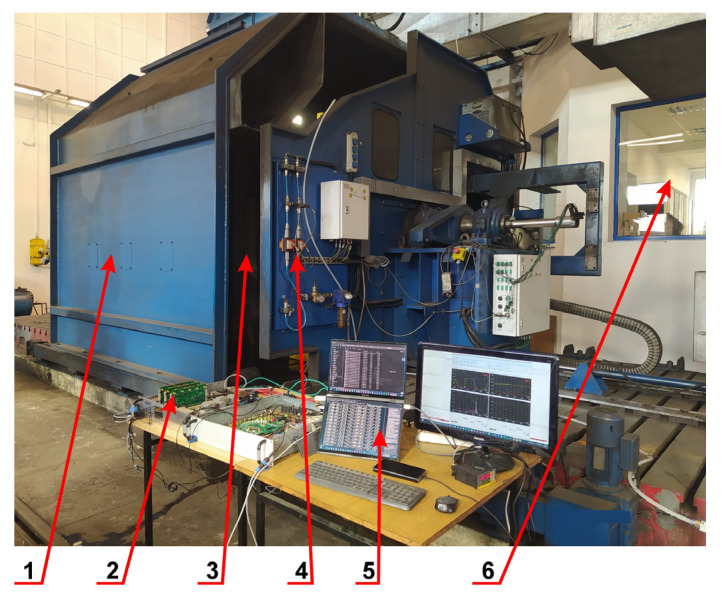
The test rig adopted for the high-speed flaw detection testing. 1—Drive system, 2—Measurement apparatus with a multichannel ultrasonic flaw detector; 3—Measuring chamber with the test wheel; 4—System for supply and measurement of the coupling fluid; 5—Computerized control and recording unit of the ultrasonic apparatus; 6—Control unit for the driving system of the test rig.
